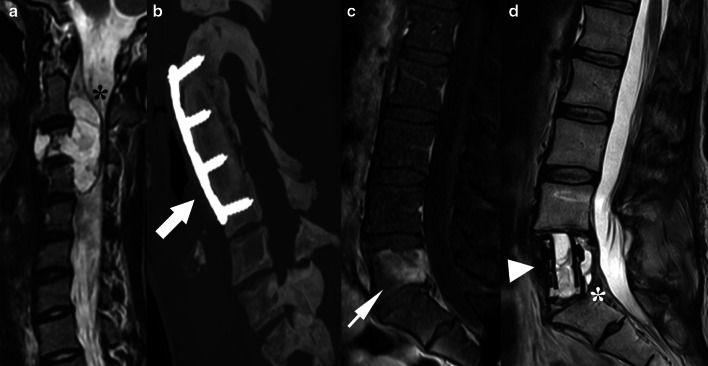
Vertebral body replacement (corpectomy). Sagittal T2W preoperative MR image (a) shows an expansile intracanal chordoma (black asterisk) centered in C3 vertebral body and extending from C2 to C4 levels. The patient underwent vertebral bodies replacement (corpectomy) and anterior fusion procedure using plate and screws (arrow), with interposition of bone graft, as shown on a postoperative sagittal using maximum intensity projection CT reconstruction in bone window (b). In another patient, sagittal T1W fat-suppressed post-contrast MR image (c) presents a spinal metastasis from breast cancer involving the L5 vertebral body (thin arrow). Postoperative sagittal T2W MR image (d) demonstrates extensive resection of L5 vertebral body (corpectomy) with placement of a spacer (arrowhead). There is a small amount of fluid around the spacer (white asterisk), in this case related to postoperative seroma that was later absorbed
